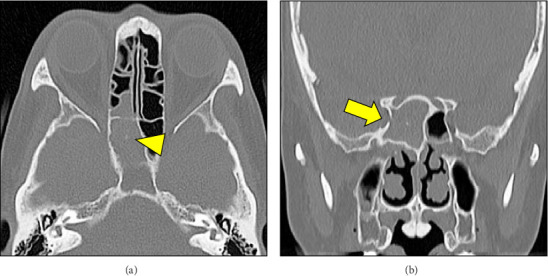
A plain computed tomography scan showing soft-tissue opacifications filling the right sphenoid sinus, calcification within the sinus (yellow arrowhead) (a), and bone thinning outside the sinus (yellow arrow) (b), raising the suspicion of mycosis fungoides of the right sphenoid sinus.
